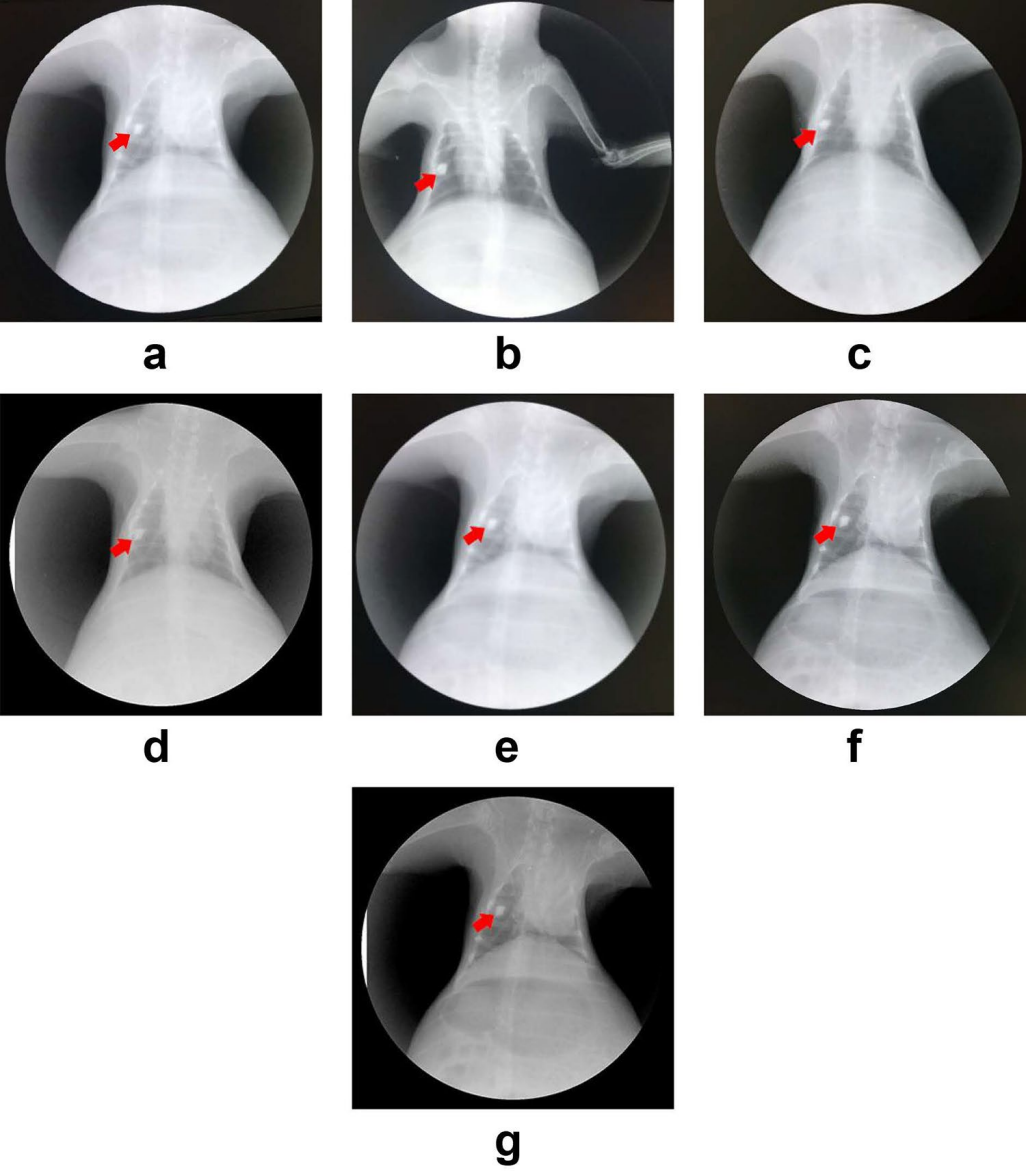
(a–g) Long-term localization of the magnetic gel in lung tissue, viewed under X-ray, are indicated by a red arrow, days 1–7 after the operation, respectively.
